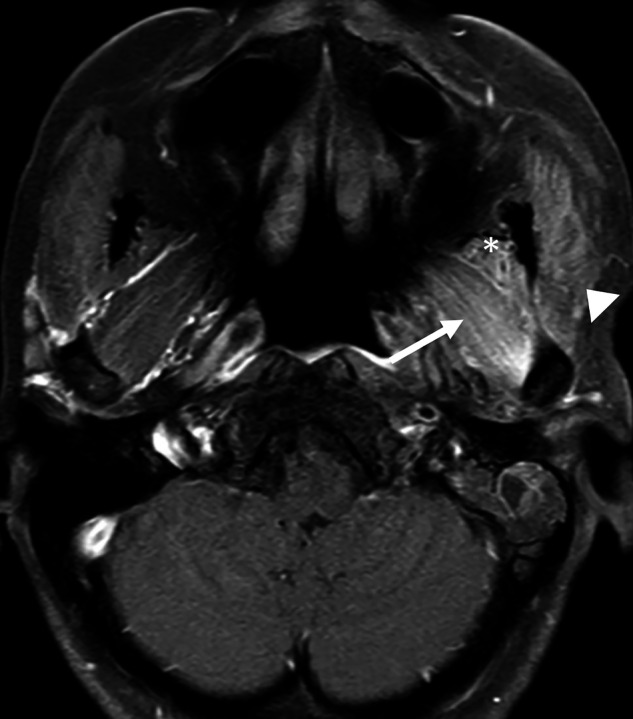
A post-treatment axial fat-suppressed contrast-enhanced T1W MRI image of a patient with left parotid myoepithelial carcinoma treated with RT 1 year ago. Radiation effects are seen in the left lateral pterygoid (white arrow), left temporalis (asterisk) and left masseter (white arrow head) muscles compared to the contralateral side
